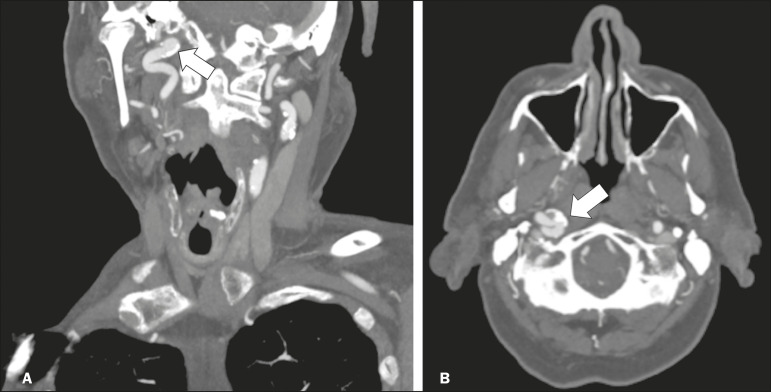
Cervical internal carotid artery aneurysm. An 82-year-old male with swallowing disturbance. CT angiography (coronal/sagittal in A; axial in B) showing a vascular loop with a partially thrombosed aneurysm in the right internal carotid artery (arrows in A and B). The lesion is along the course of the hypoglossal nerve.
